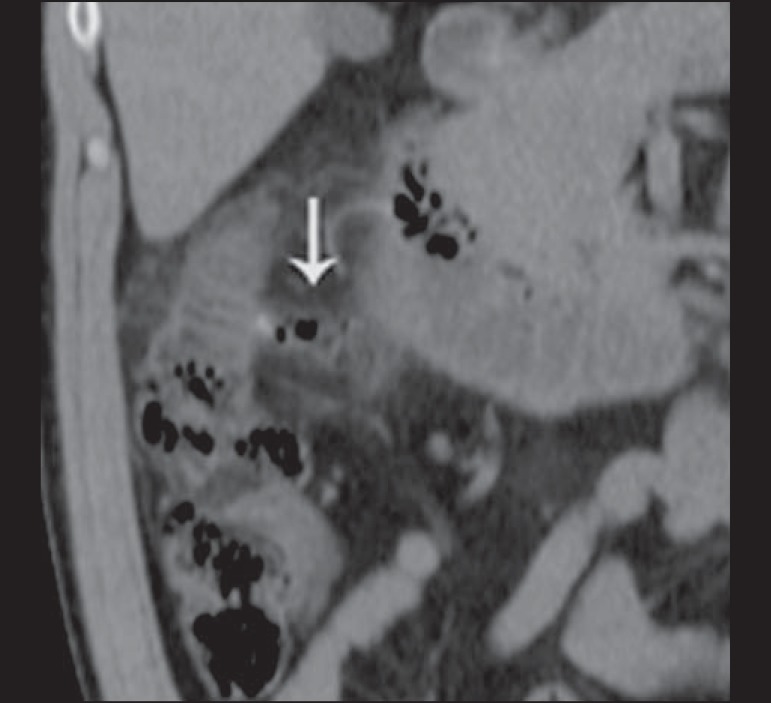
Hinchey stage Ia. Abdominal CT, in the coronal plane, without contrast.
